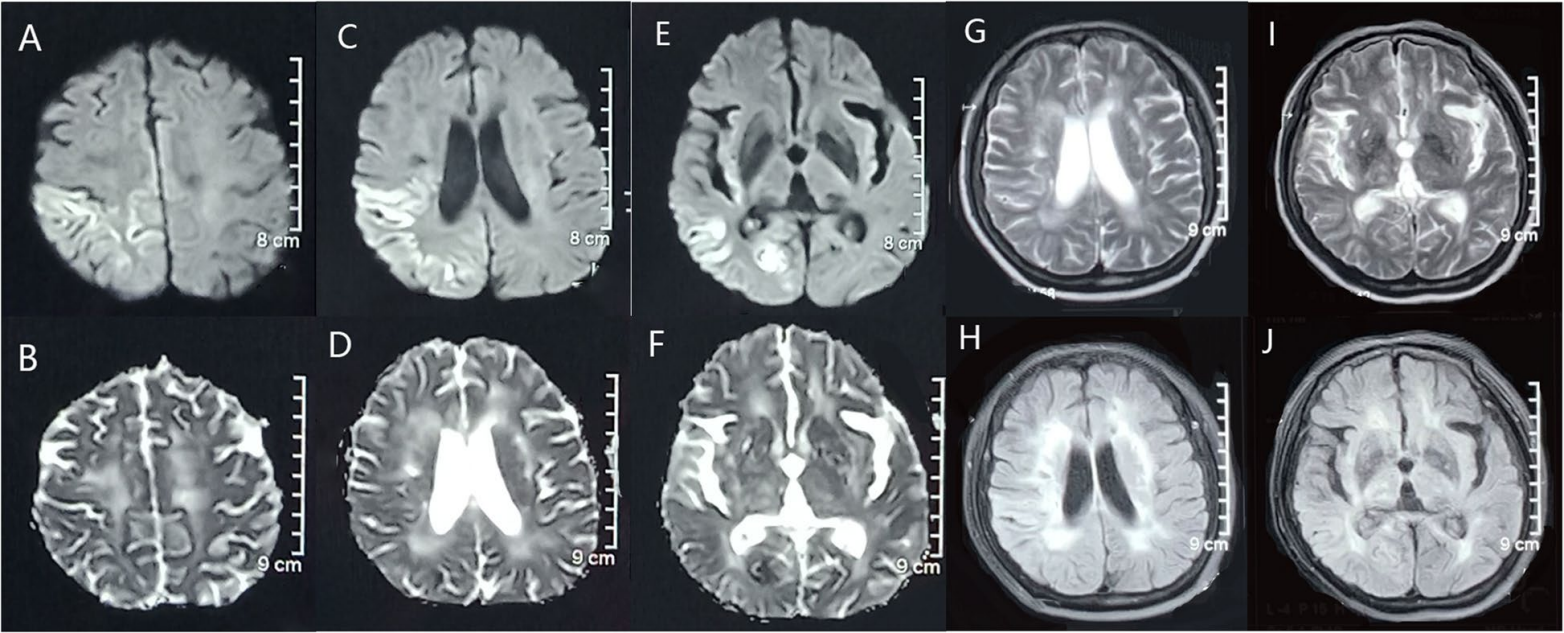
Brain MRI of the patient in August 2019 DWI showed the high signal in the cortices of right parietal lobe, right temporal lobe and right occipital lobe (A, C, E) with corresponding decreased ADC values (B, D, F). Brain MRI showed multiple ischemic foci and softened foci on T2 image (G, I), and extensive white matter lesions on FLAIR image (H, J)
